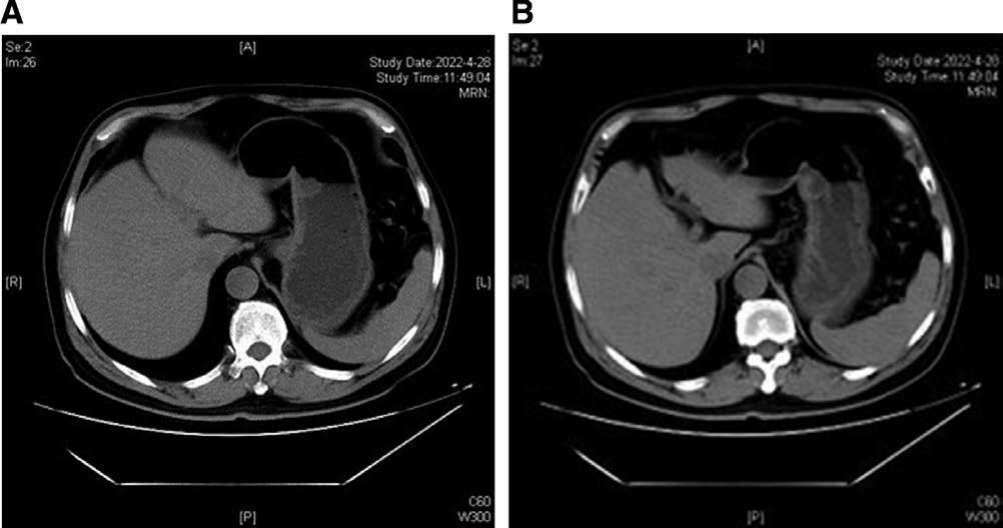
CT findings. (A and B) Abdominal CT showed a round low-density shadow in the small curvature of the stomach. CT = computed tomography.
